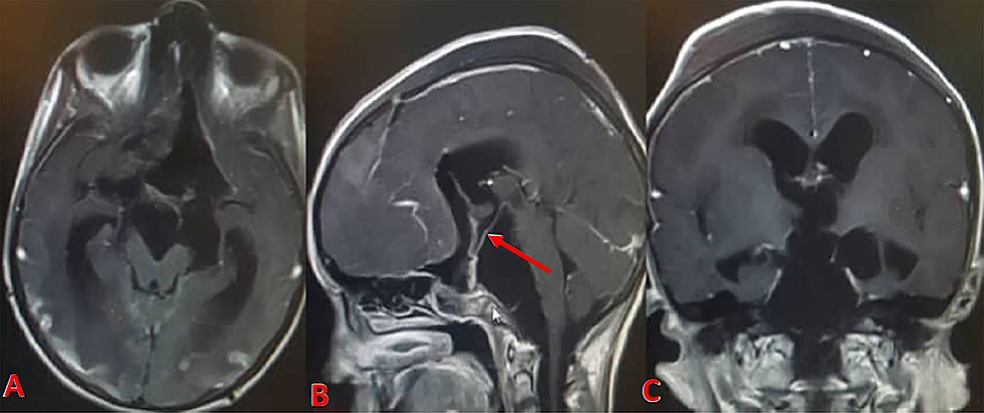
Follow-up MRI at two years Axial (A), sagittal (B), and coronal (C) postcontrast T1-weighted images revealing no re-accumulation of cystic contents. The elongated infundibulum of the pituitary gland is noted (arrow in B).
